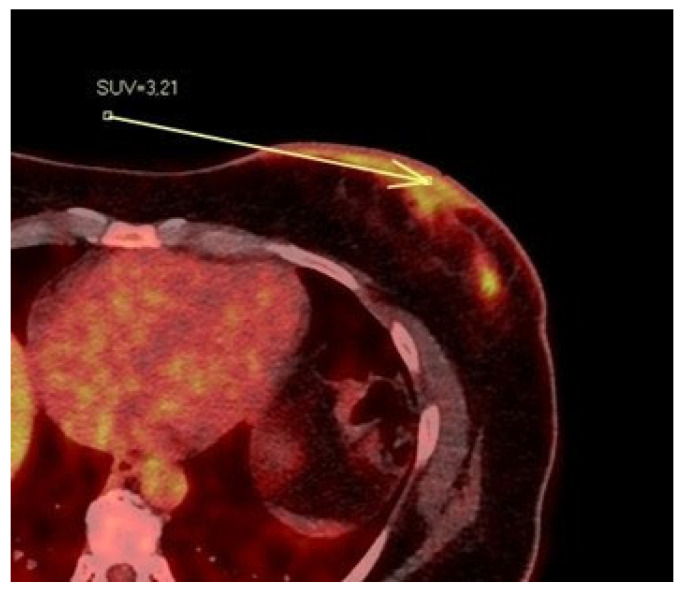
Patient’s name R.M. During PET/CT, the pathological NAC-SUV value is measured at the base of the left nipple.
